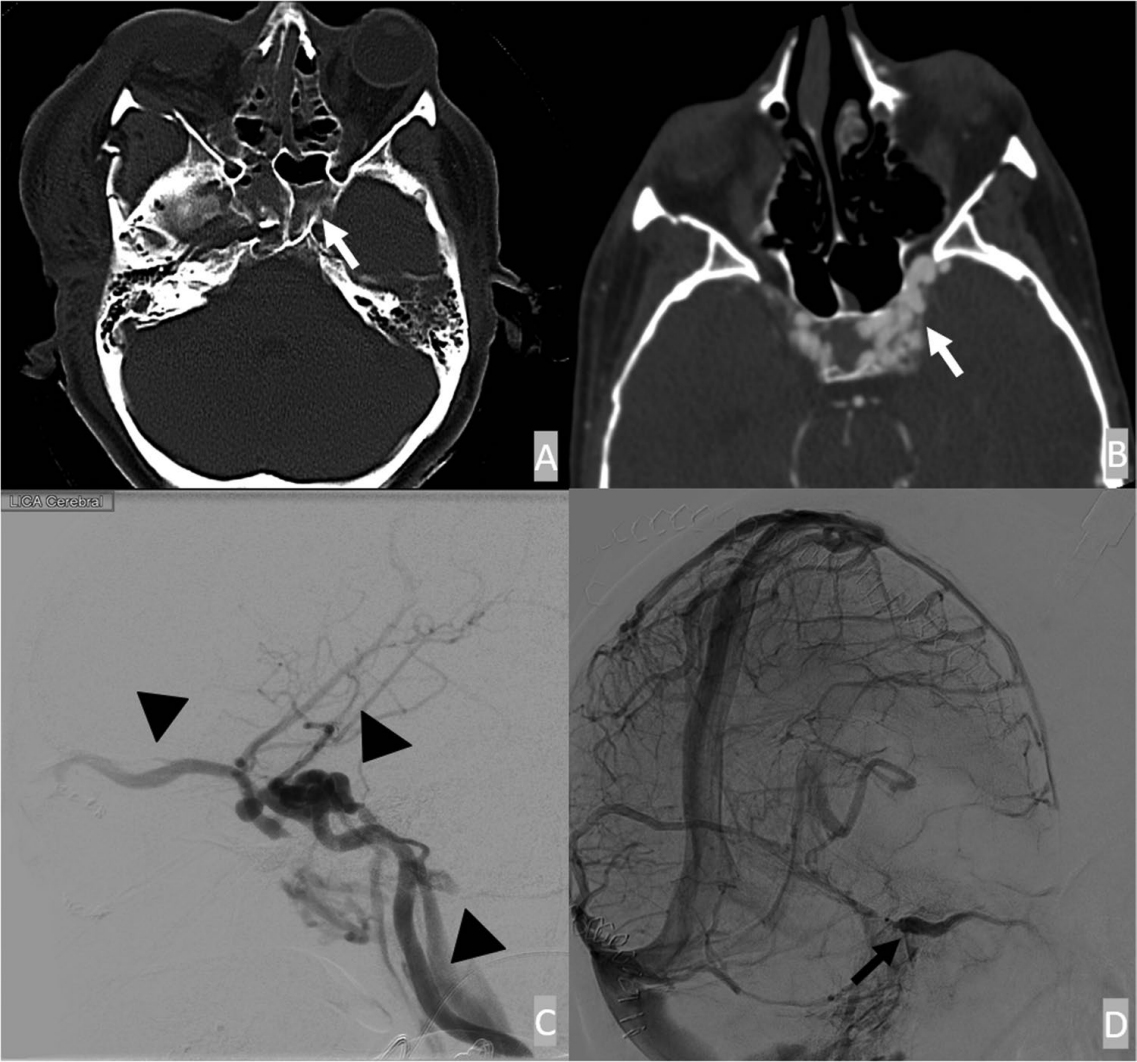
Carotid-cavernous fistula (CCF). A 47-year-old female presented after a motor vehicle collision with extensive craniofacial fractures including a fracture involving the left sphenoid sinus wall (A). Four months later, the patient presented with pulsatile tinnitus and exophthalmos. Axial CT head angiogram (B) demonstrated bulging and asymmetric enhancement of the left cavernous sinus, compatible with a carotid-cavernous fistula. The patient subsequently underwent DSA. The lateral projection of arterial phase (C) showed shunting from the left internal carotid artery to the cavernous sinus and the enlarged draining veins. There was retrograde flow from the cavernous sinus into the ophthalmic vein (arrowheads). The venous phase of the internal carotid artery in oblique projection (D) demonstrated prolonged filling of the superior ophthalmic vein (black arrow)
